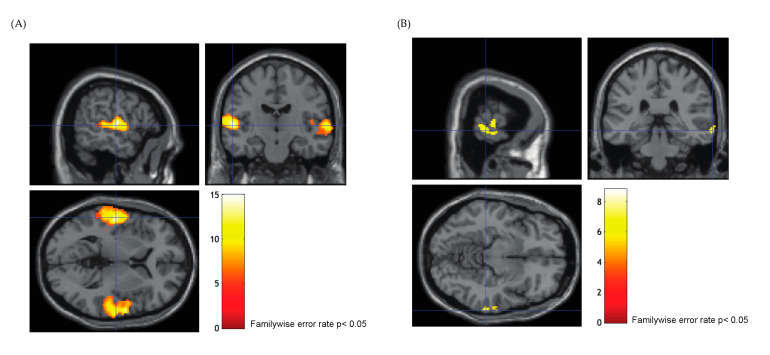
Functional magnetic resonance imaging activation in the narrative forward condition. The crosshair line on the images indicates the coordinate of the local maxima. (A) Significant activation distributed over the primary auditory cortex and superior temporal gyrus was found bilaterally in P1 (familywise error rate *p* < 0.05). (B) Unilateral activation was found in three patients (P5, P7, and P16), and auditory cortex activation appeared primarily in the right hemisphere in P16 (familywise error rate *p* < 0.05).
